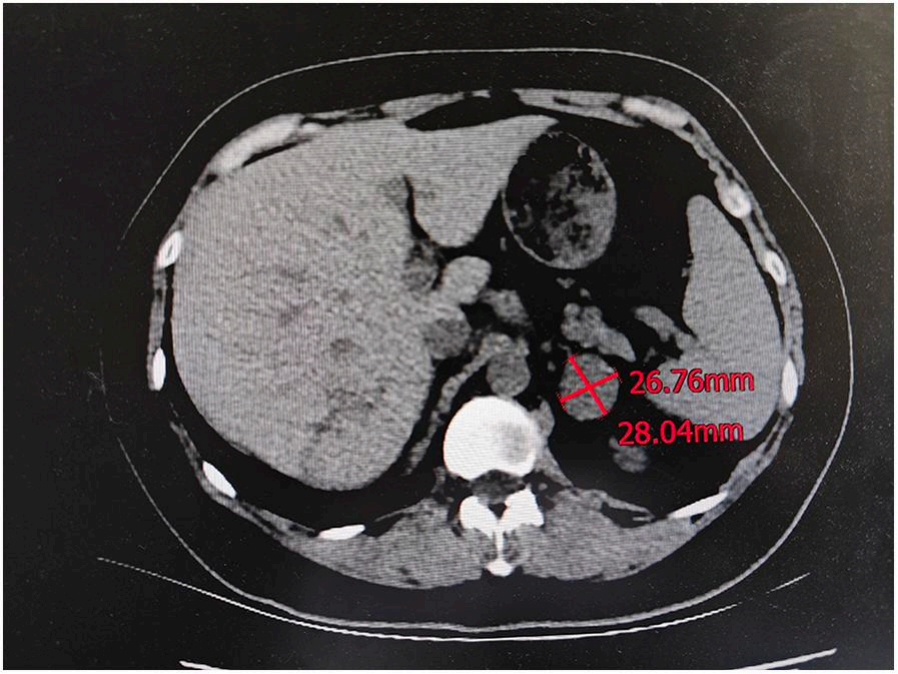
Computed tomography (CT) of the abdomen. CT of the abdomen shows a left adrenal mass measuring 2.8 cm by 2.6 cm.
